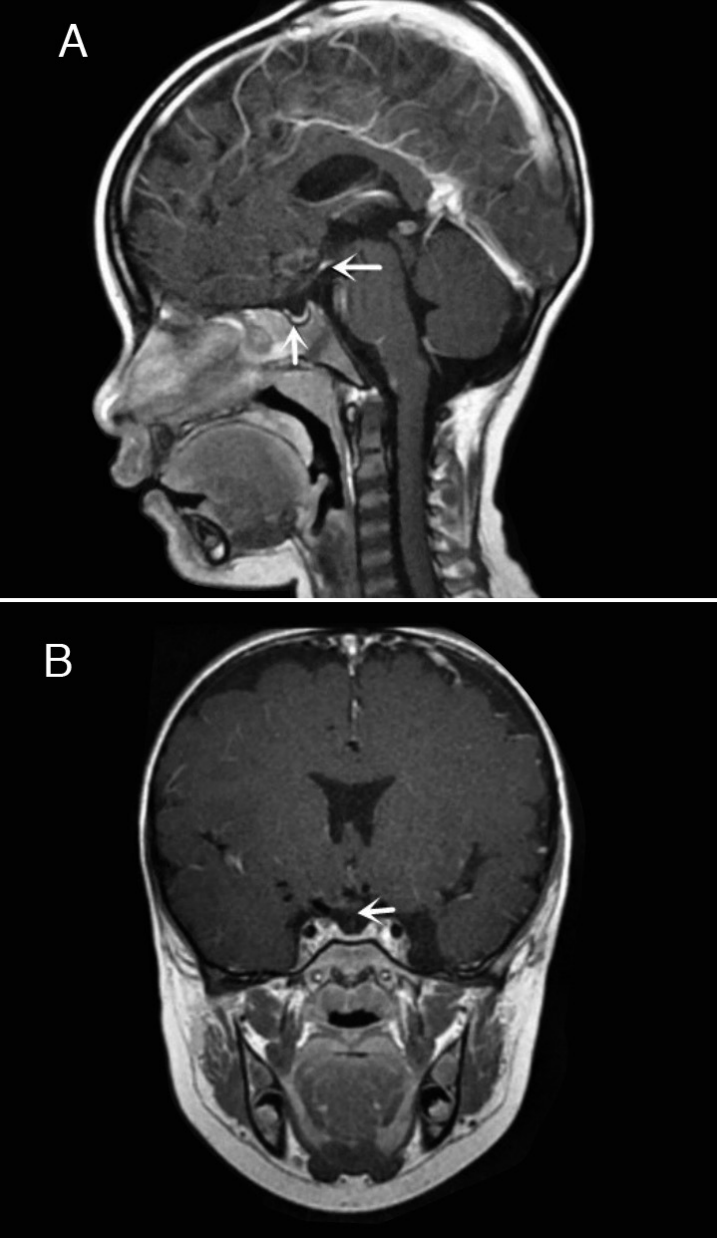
Sagittal and coronal magnetic resonance imaging of the pituitary confirming pituitary stalk interruption syndrome (A) Sagittal view. The small anterior pituitary (vertical arrow) and the posterior lobe was localized at the hypothalamic region (horizontal arrow). (B) Coronal view. The pituitary stalk is absent
